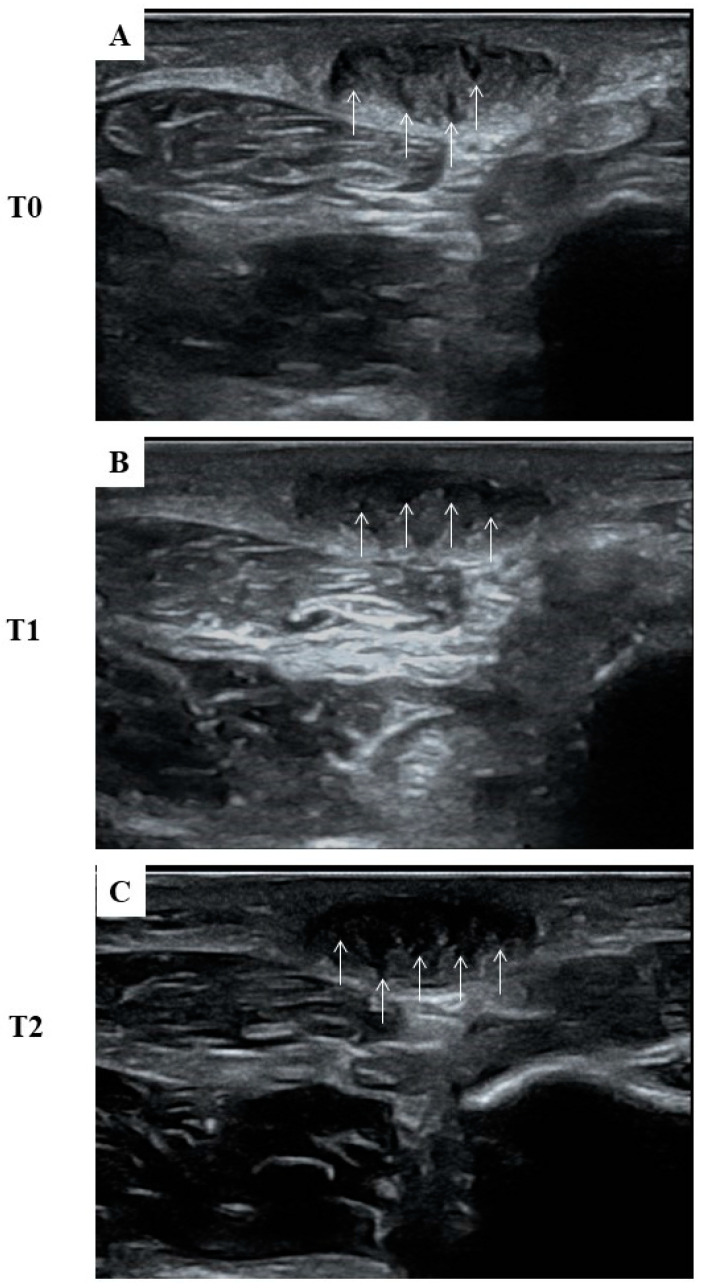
Transverse ultrasonographic images of the left symptomatic nodule at different time intervals. (A) Pre-treatment (T0) US examination of the symptomatic nodule revealed a distinctive intralesional feature known as the “comb sign”. This latter, featured by an alternating dark and light linear band, is also evident at (B) one week (T1) and (C) two months (T2) after ESWT treatment. It can be noted that the dark bands (arrows) within the nodule become progressively larger than the light ones from T0 to T2.
